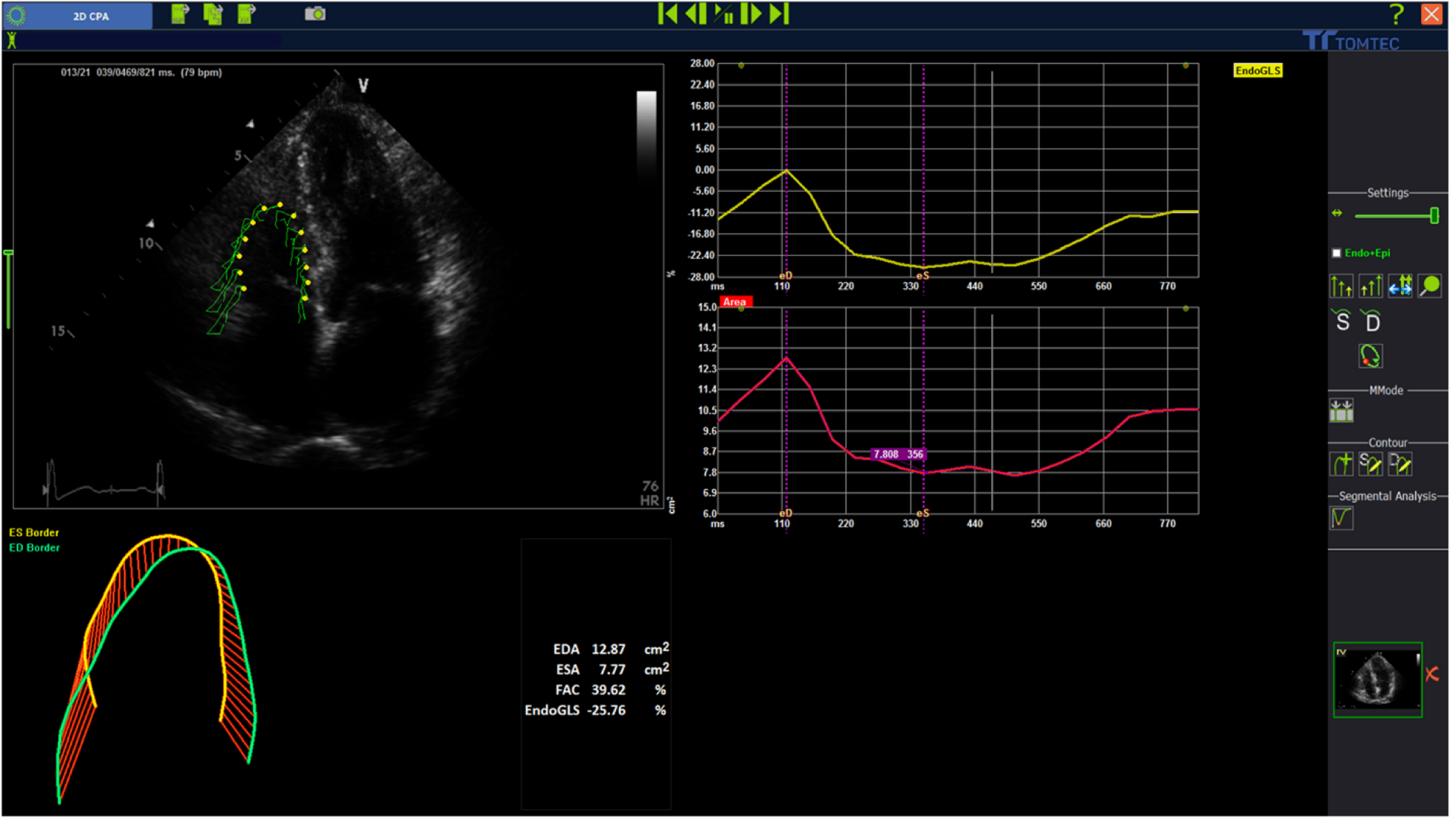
RVFAC obtained by the TomTec software (2D cardiac performance analysis) 2D speckle-tracking echocardiography. FAC was measured using RV end-diastolic area (RVDA) and end-systolic area (RVSA) obtained by manual tracing of the RV endocardium at end-diastole and end-systole in the apical 4-chamber view. The formula: [RVDA-RVSA/RVDA] × 100 was then used to calculate FAC. To limit sub-optimal interobserver reproducibility, it was ensured that the RV was contained in the whole imaging frame throughout systole and diastole, while ensuring that trabeculae in RV cavity was included. LVEF: Left ventricular ejection fraction. RVFAC: Right ventricular fractional area change. RVFWS: Right ventricular free wall strain. RVGLS: Right ventricular global longitudinal strain
